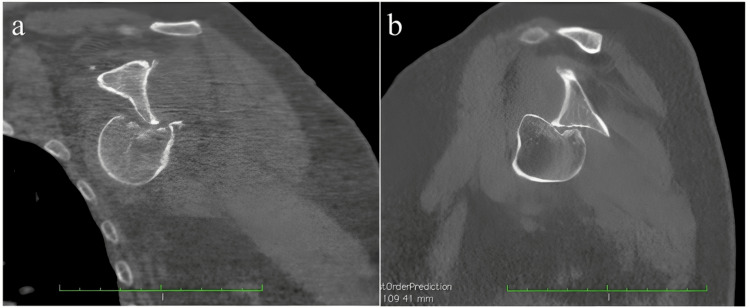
Computed tomography of the left shoulder.
